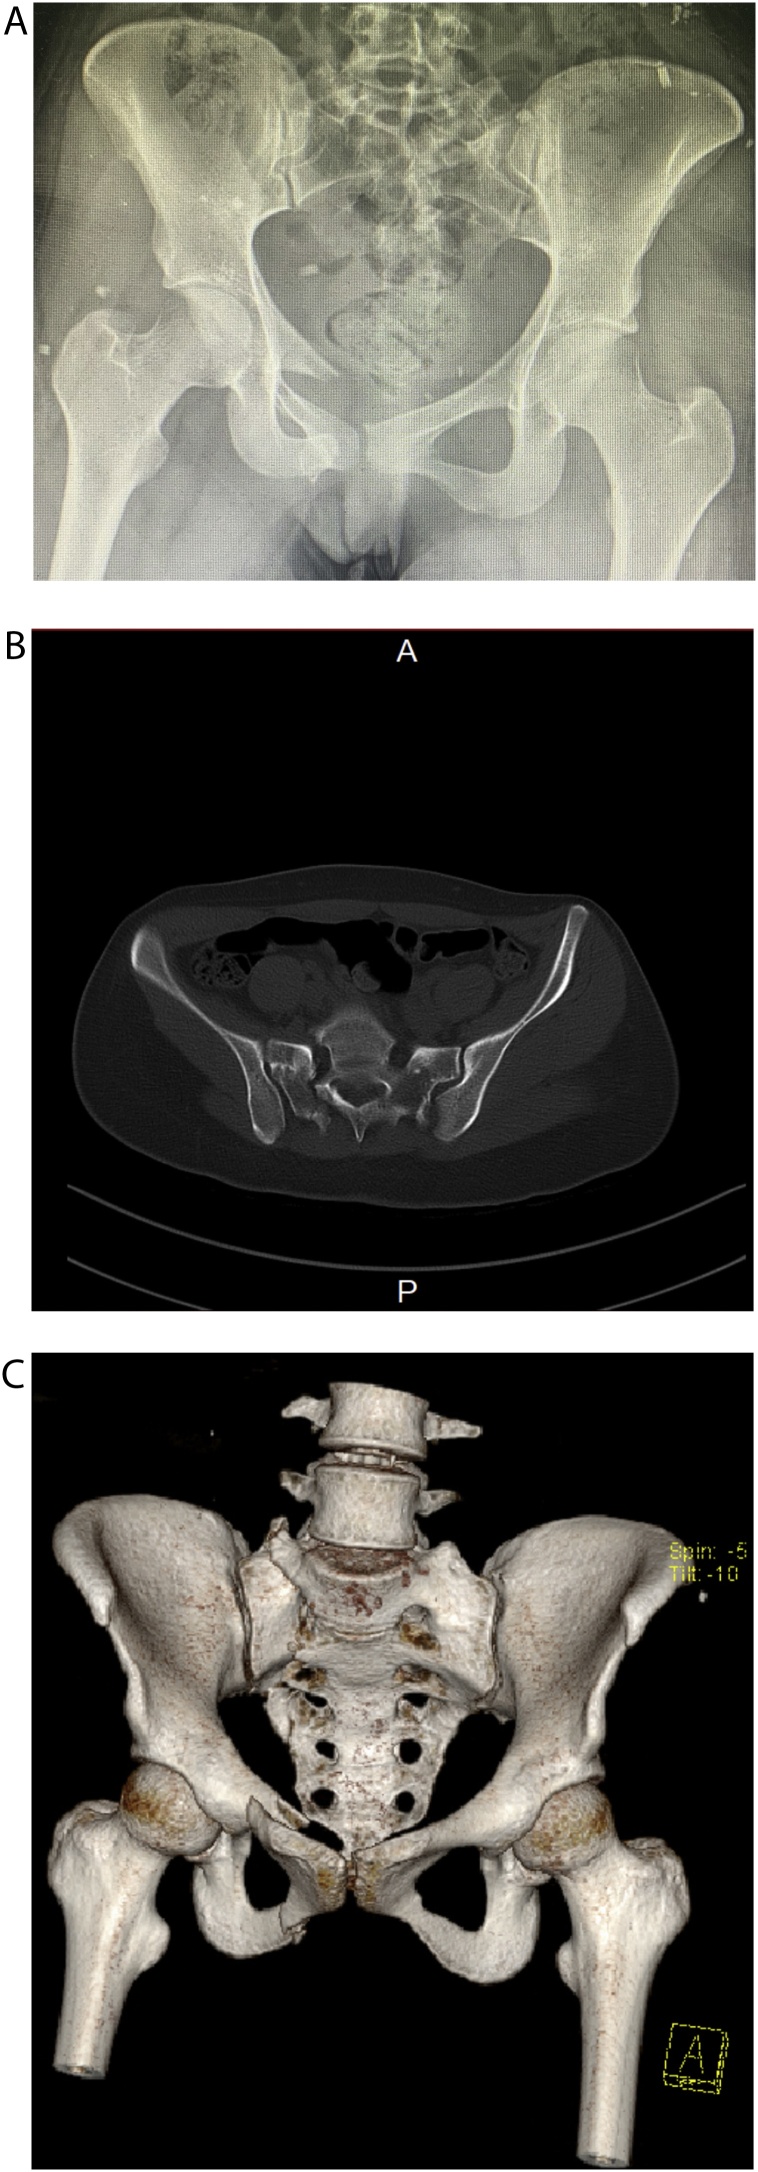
A: Anteroposterior pelvis view shows suspicious vertical displacement of right hemipelvis due to right sacral fracture, and right anterior ramus fracture. B: CT (axial cut), and C (3D reconstruction image); delineates clearly that the fracture is transforaminal, and is displaced posteriorly, no vertical displacement is identified based on CT images.
